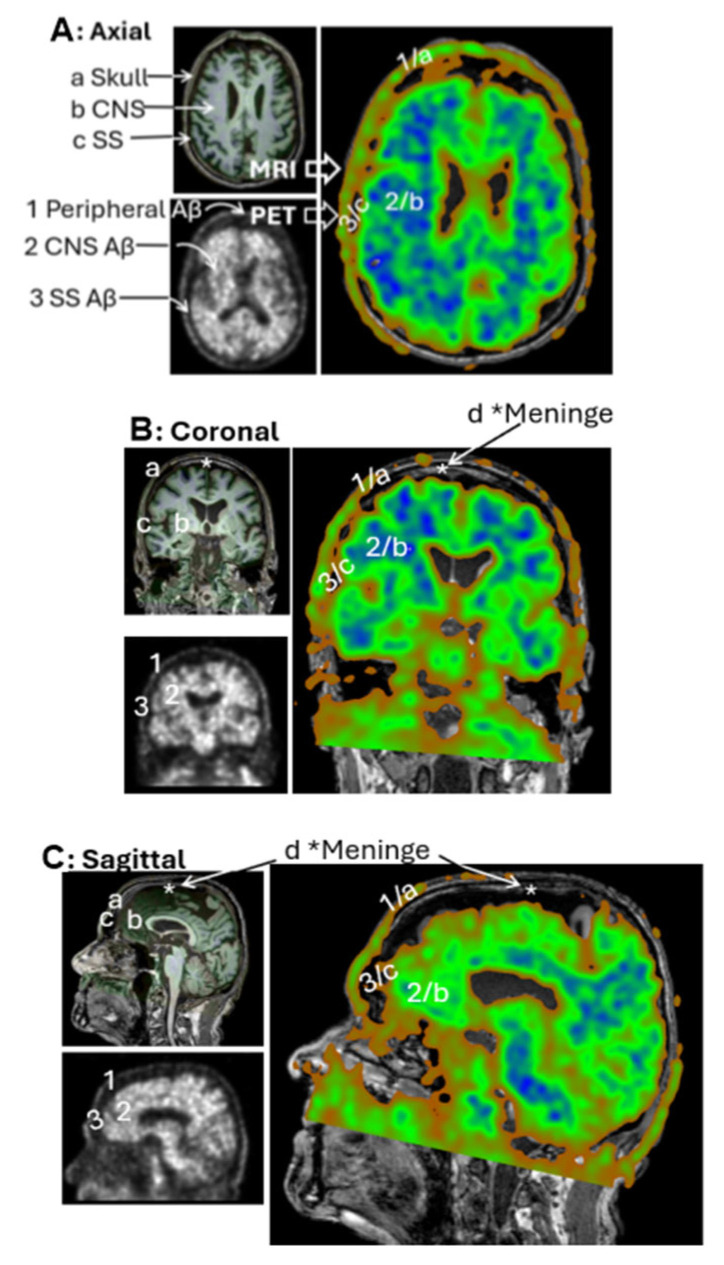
PET/MRI Coregistration. Representative MRI (left top panels) and PET scans (left bottom panels) from the CU-3 individual. Coregistration was conducted with 3D Slicer to set PET as the moving image (blue-green right panels) and MRI as the fixed reference (dark-gray right panels). Anatomical regions identified on MRI include a—Skull, b—Central nervous system (CNS), c—Subarachnoid space (SS), d—Meninges. Corresponding regions identified on PET include 1—extracranial Aβ signal, 2—CNS signal, 3—SS signal. Subpanels: (A) Axial view, (B) Coronal view, (C) Sagittal view. Extracranial Aβ signals are spatially aligned with the skull, not the meningeal regions.
